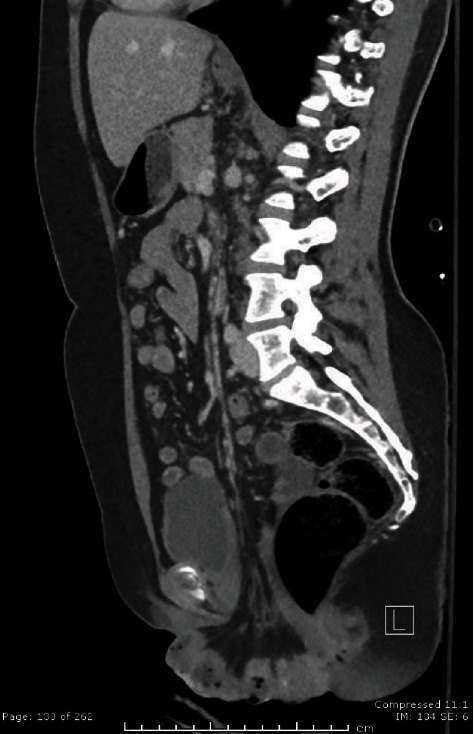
Axial CT image with intravenous contrast: image demonstrates pelvic floor dysfunction with rectovaginal space widening.
